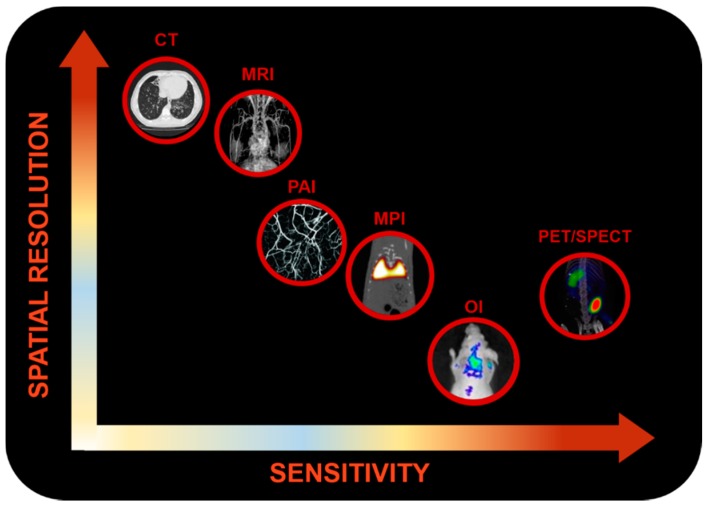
Graphical representation of different molecular imaging (MI) techniques classified by their sensitivity and spatial resolution. Computed tomography (CT) image adapted with permission from Reference [27]. Photoacoustic imaging (PAI) image adapted with permission from Reference [28]. Magnetic particle imaging (MPI) image adapted with permission from Reference [22]. Optical imaging (OI) image adapted with permission from Reference [29]. Positron-emission tomography (PET) image adapted with permission from Reference [30].
